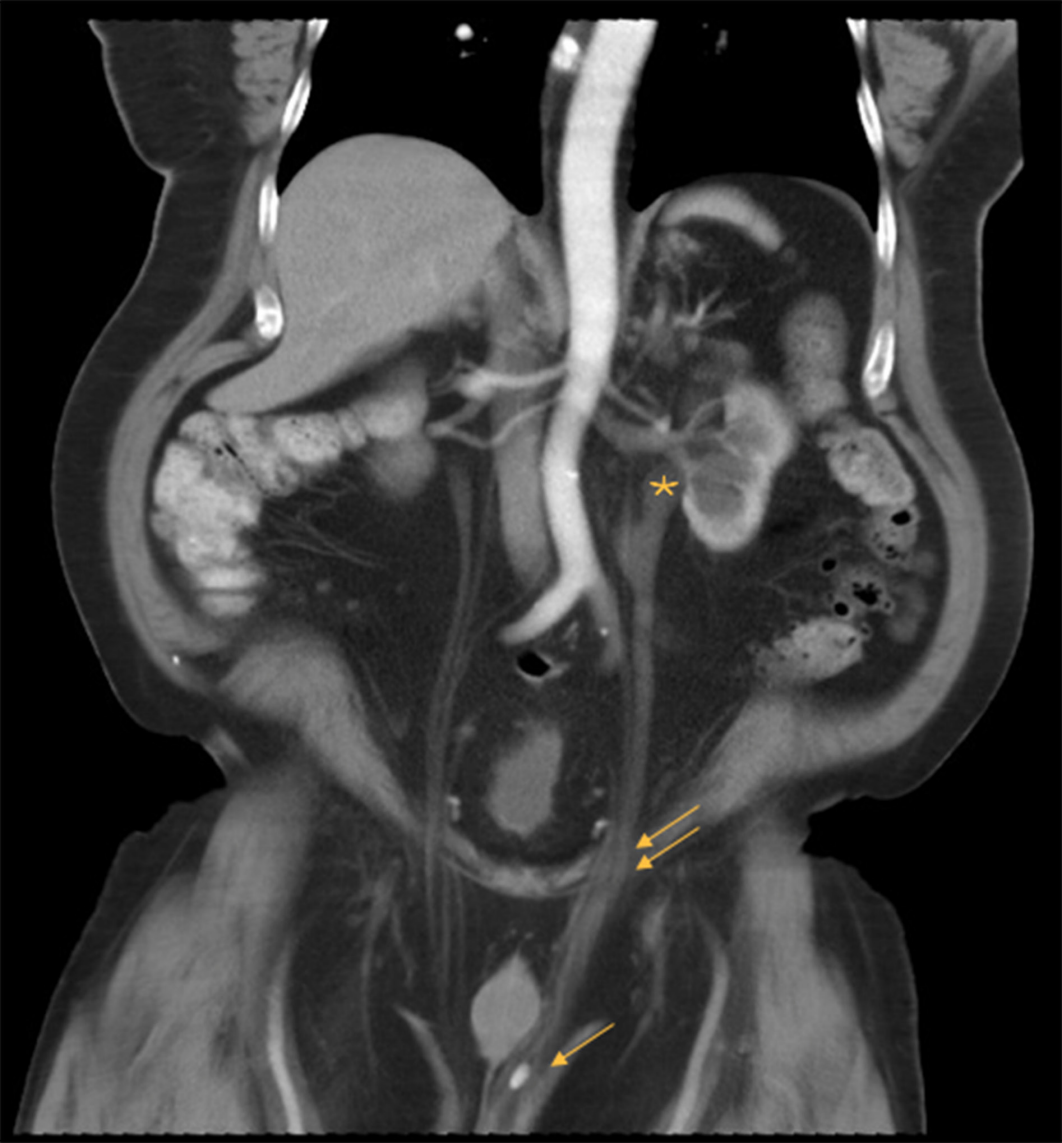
Coronal contrast-enhanced CT in nephrographic phase demonstrating left hydroureteronephrosis (*). Dilated left ureter noted to pass through inguinal canal (double arrows) and into the left hemiscrotum containing a stone (single arrow).
